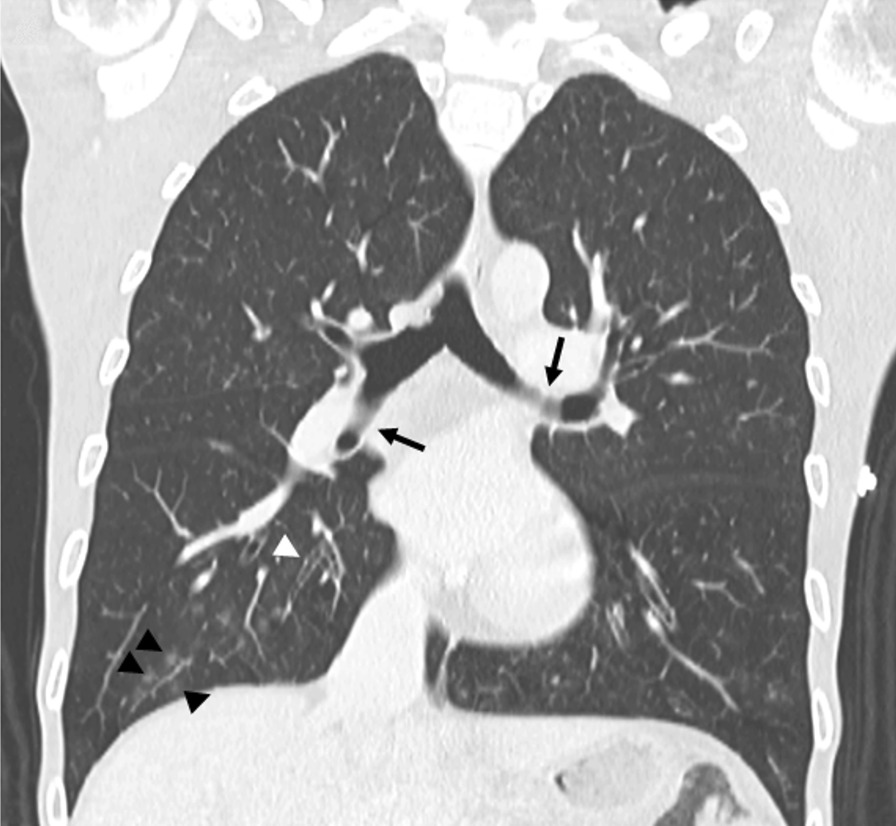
Chest computed tomography showing mucus hypersecretion (black arrows), thickening of the peri-bronchiolar walls (white arrowheads) and a “tree-in-bud” pattern reflecting bronchiolar mucoid impaction with additional involvement of adjacent alveoli (black arrowheads)
